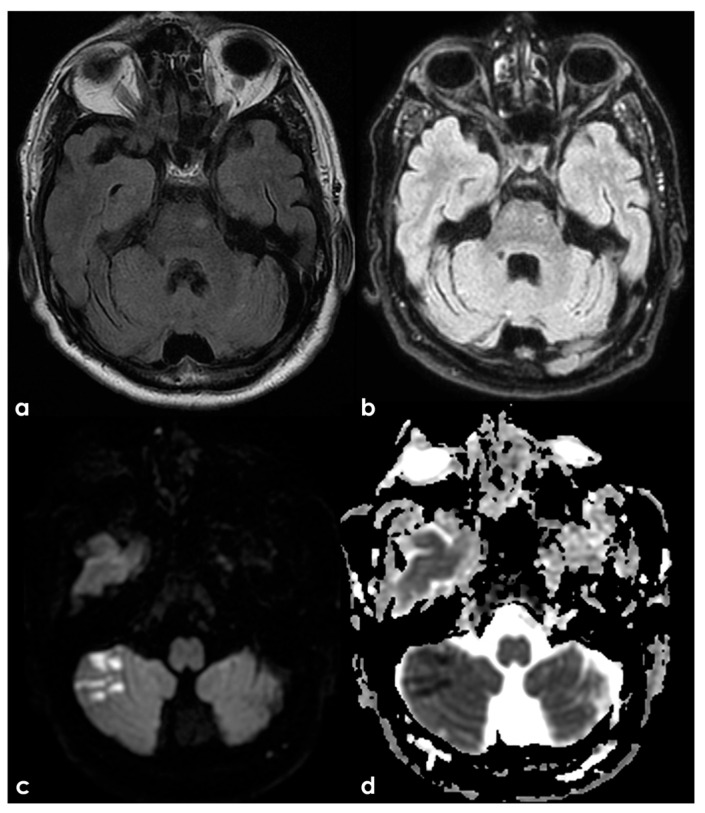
Brain Magnetic Resonance Imaging (MRI) of the same patient referenced in Figure 1. Panel (a) and (b) show axial Fluid Attenuated Inversion Recovery (FLAIR) sequence ten and thirty days after ischemic stroke, respectively, highlighting a left pontine small subcortical infarction (SSI) (panel (a)) that rapidly evolved in a cavitated lesion (panel (b)), confirming the proposed timing. In panel (c,d), Diffusion Weighted Imaging (DWI) and Apparent Diffusion Coefficient (ADC) show a right multifocal cerebellar acute ischemic lesion.
